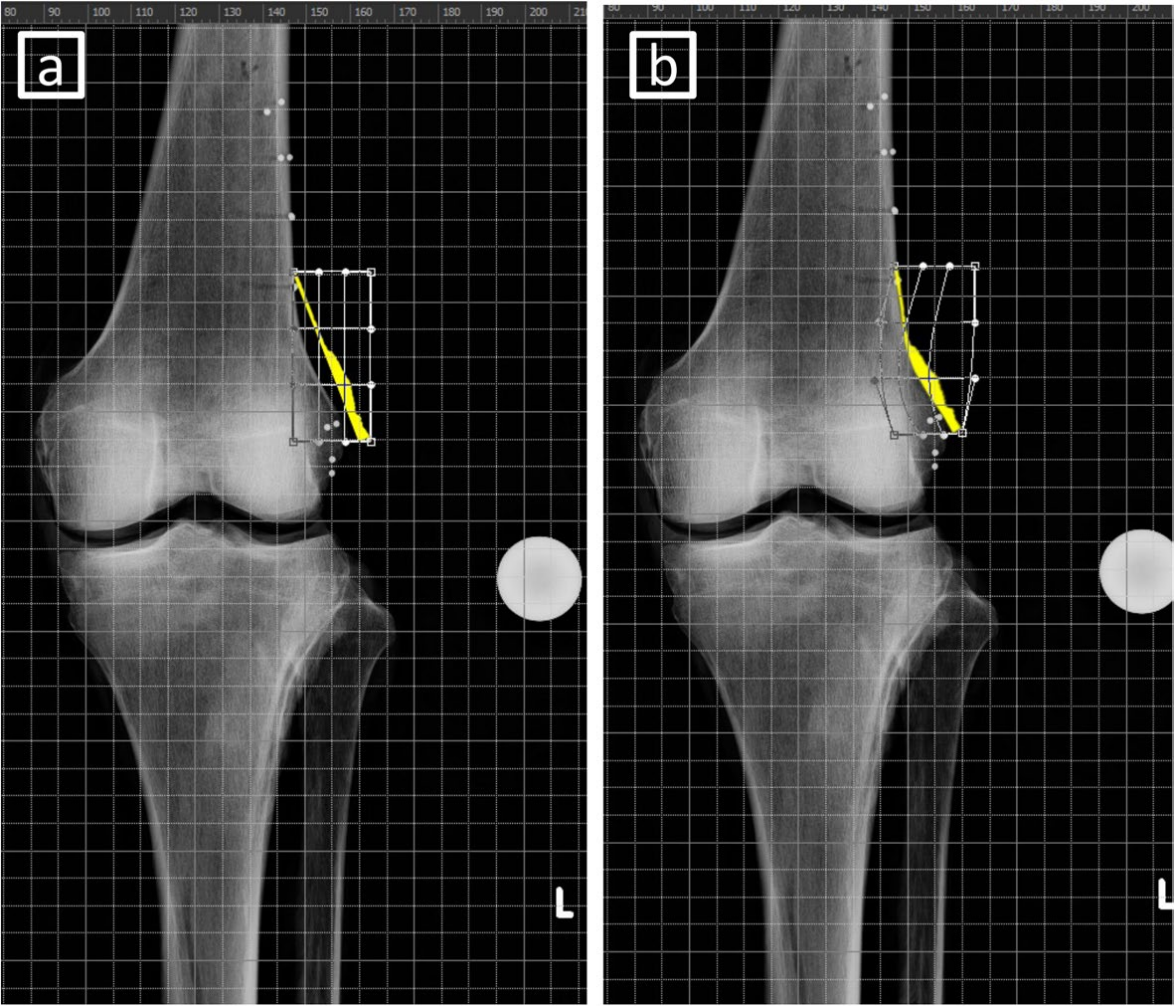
Methods of overlaying the graph of periosteum with X-ray image by image editing software. a Before the deformation. The most distal part of the graph was placed at the level of the lateral epicondyle and parallel to the curved part of the femoral condyle. b After the deformation. The graph was deformed along the curved part of the metaphysis of the distal femur
